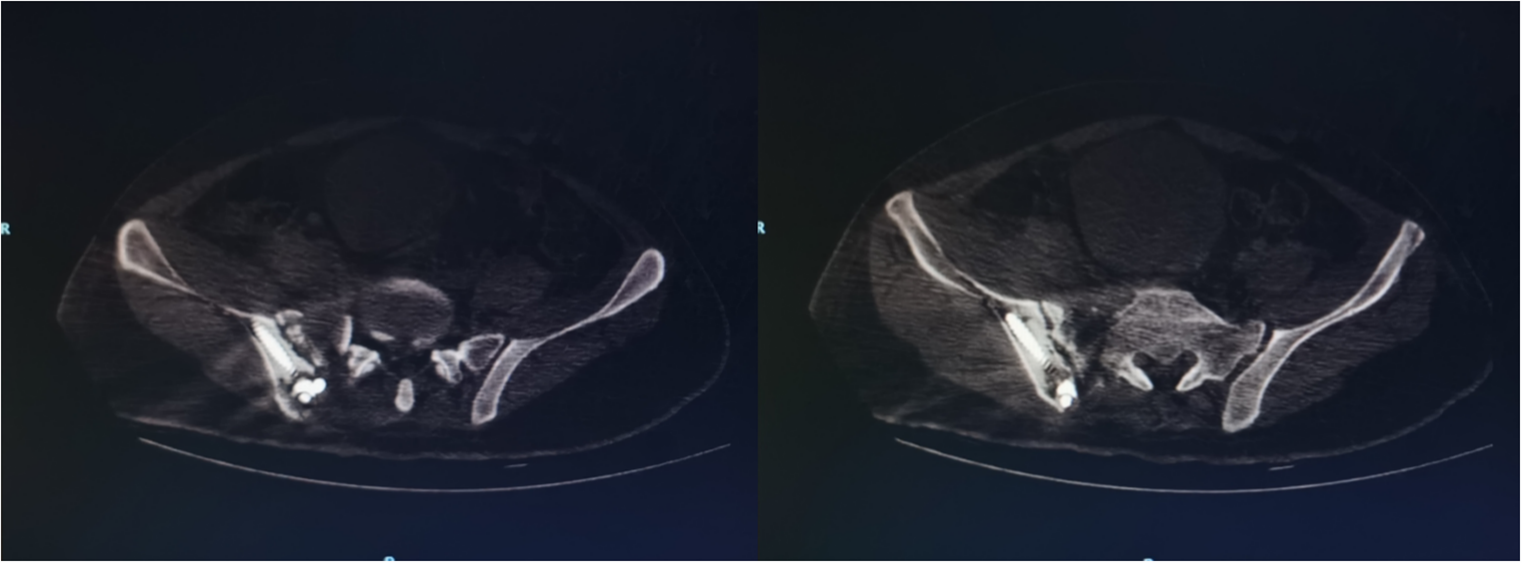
CT scan showed that the iliac screws was in good position and did not puncture the iliac cortex
